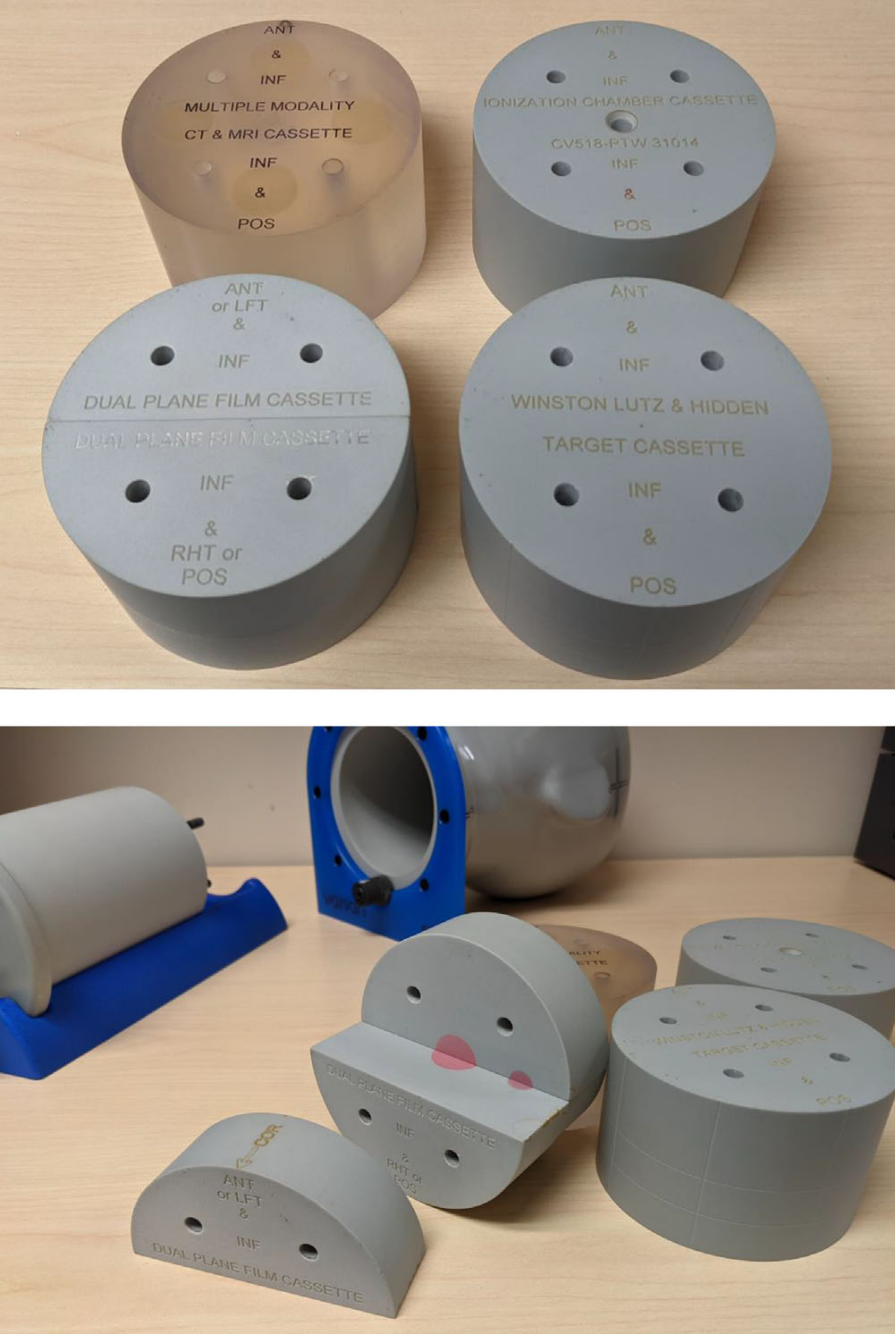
Target cassettes used for dosimetric and geometric quality assurance. (Top) Four types of target cassette, clockwise starting upper left: multiple‐modality computed tomography (CT) and magnetic resonance imaging (MRI), ionization chamber, Winston–Lutz/hidden target, and dual‐plane film cassettes. (Bottom) One of the four segments that comprises the film cassette has been removed to show the contrast‐enhanced (pink) targets used for planning
